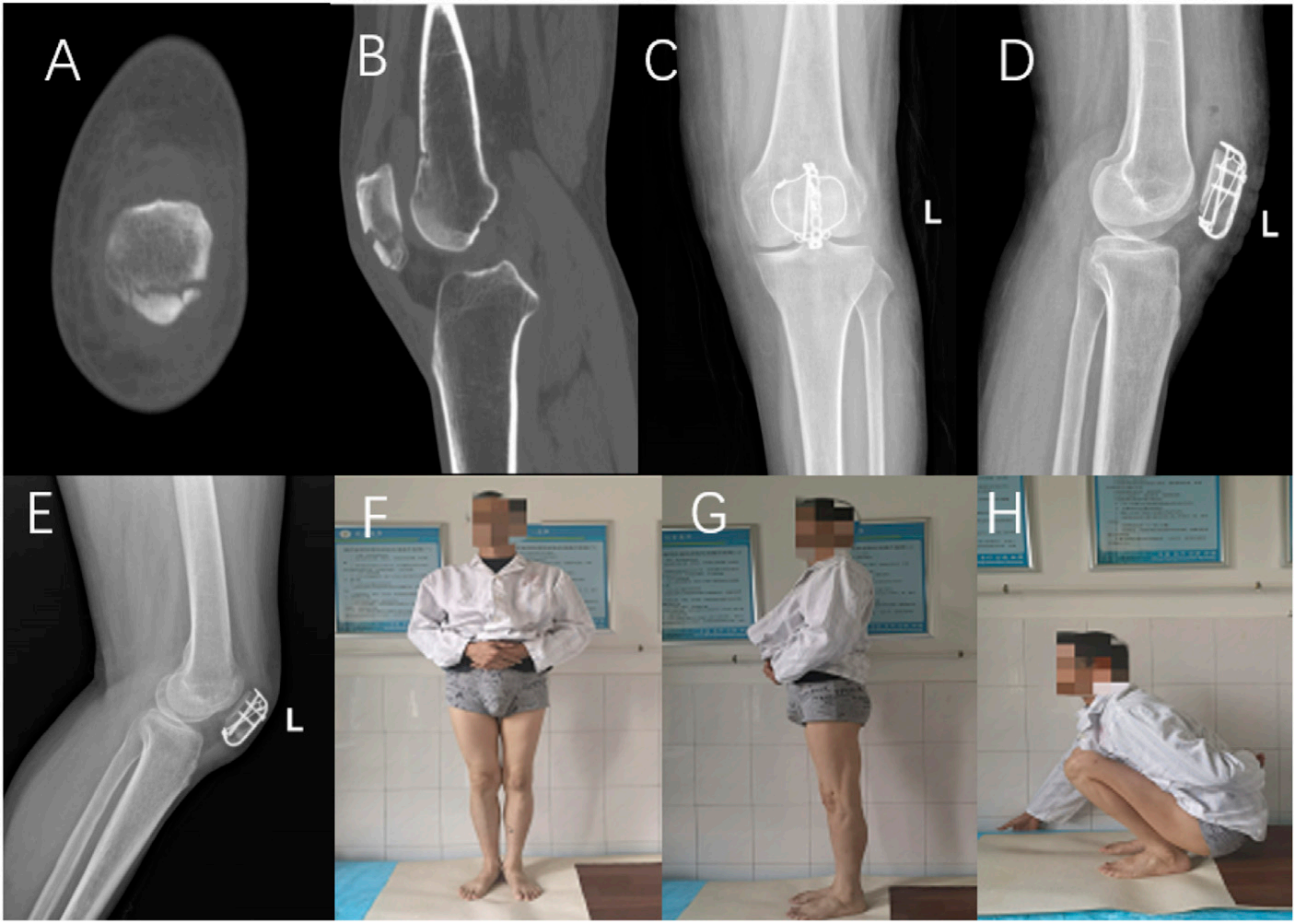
A 56-year-old male presented with a fracture of the inferior pole of the left patella caused by a fall injury. Preoperative CT (A,B), postoperative knee X-ray (C,D). X-ray films and knee joint function 12 months after operation (E–H).
